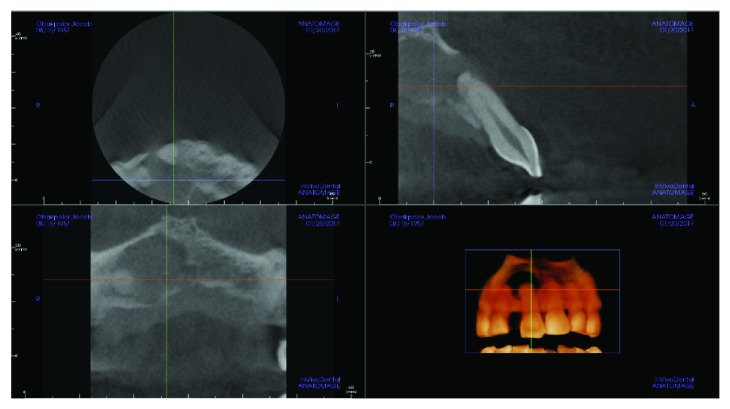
CBCT image showing large cystic-appearing defect in the anterior maxilla with perforation of the buccal and palatal cortices.
